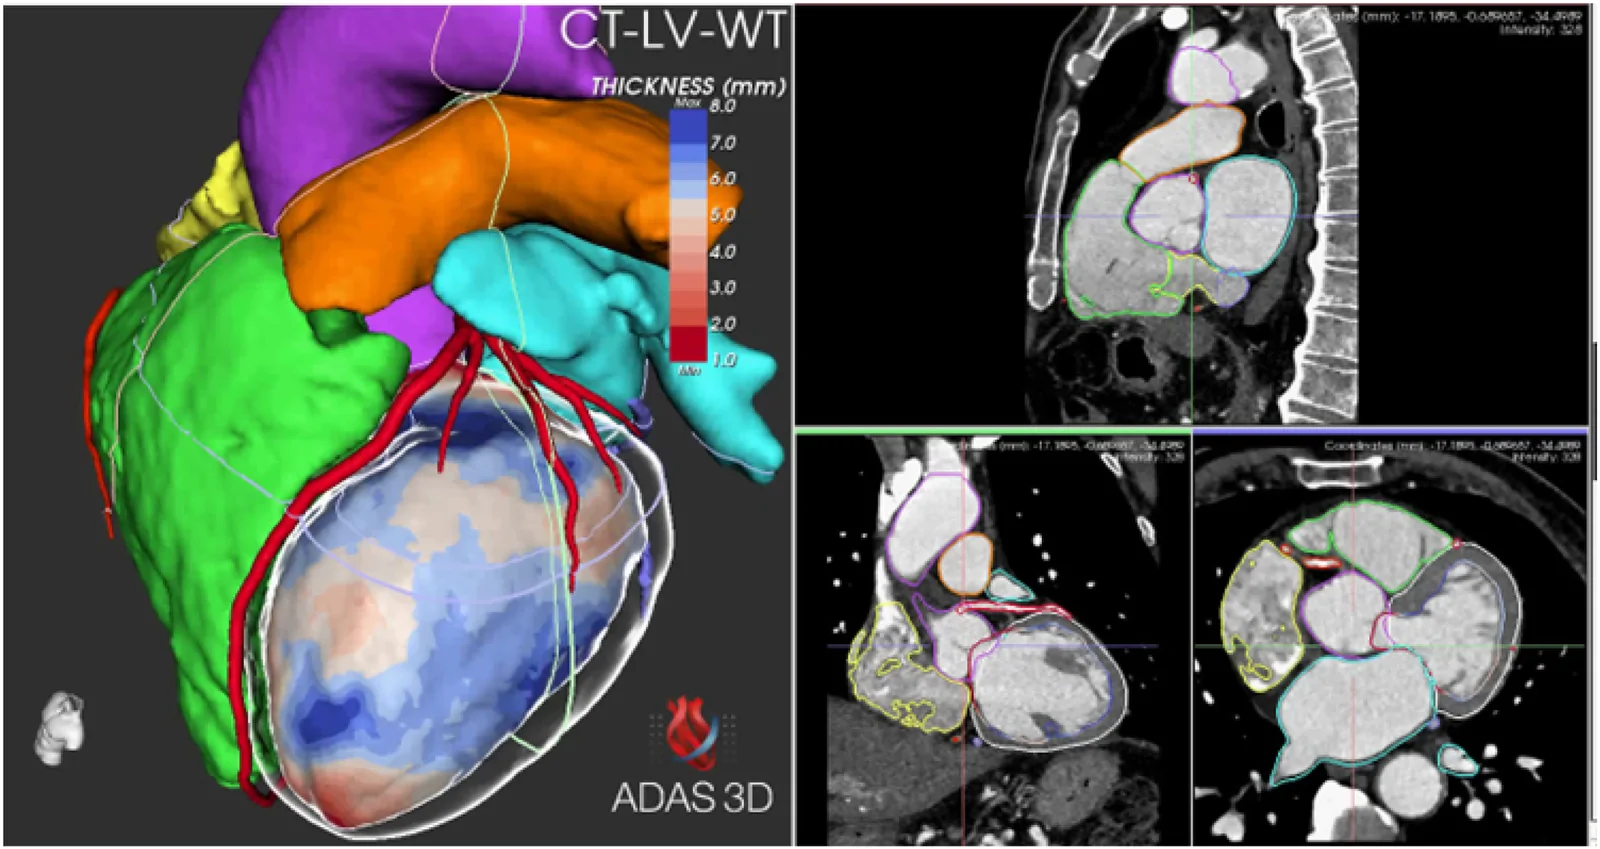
LV wall thickness derived from CT (Image courtesy of ADAS 3D Medical SL, Barcelona, Spain.).
